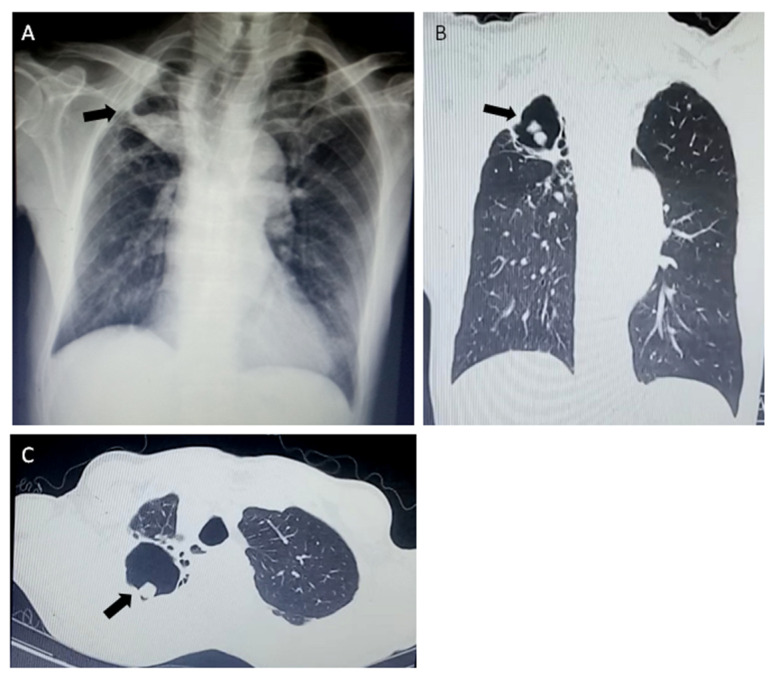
Radiological findings of chronic pulmonary aspergillosis (CPA) in a 51-year-old male patient two months after completion of TB treatment. A large cavity with aspergilloma (black arrows) of the left upper lobe in chest X-ray (A) and computed tomography scans (B,C).
